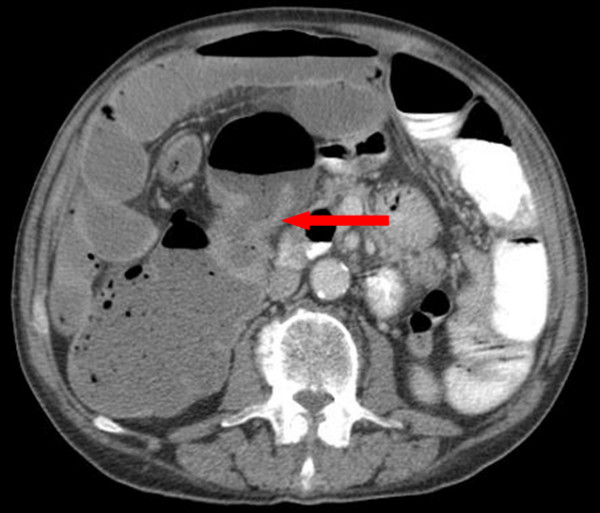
Imaging of the abdomen (cross sectional). CT scan showing tumour of the ascending colon (red arrow).
